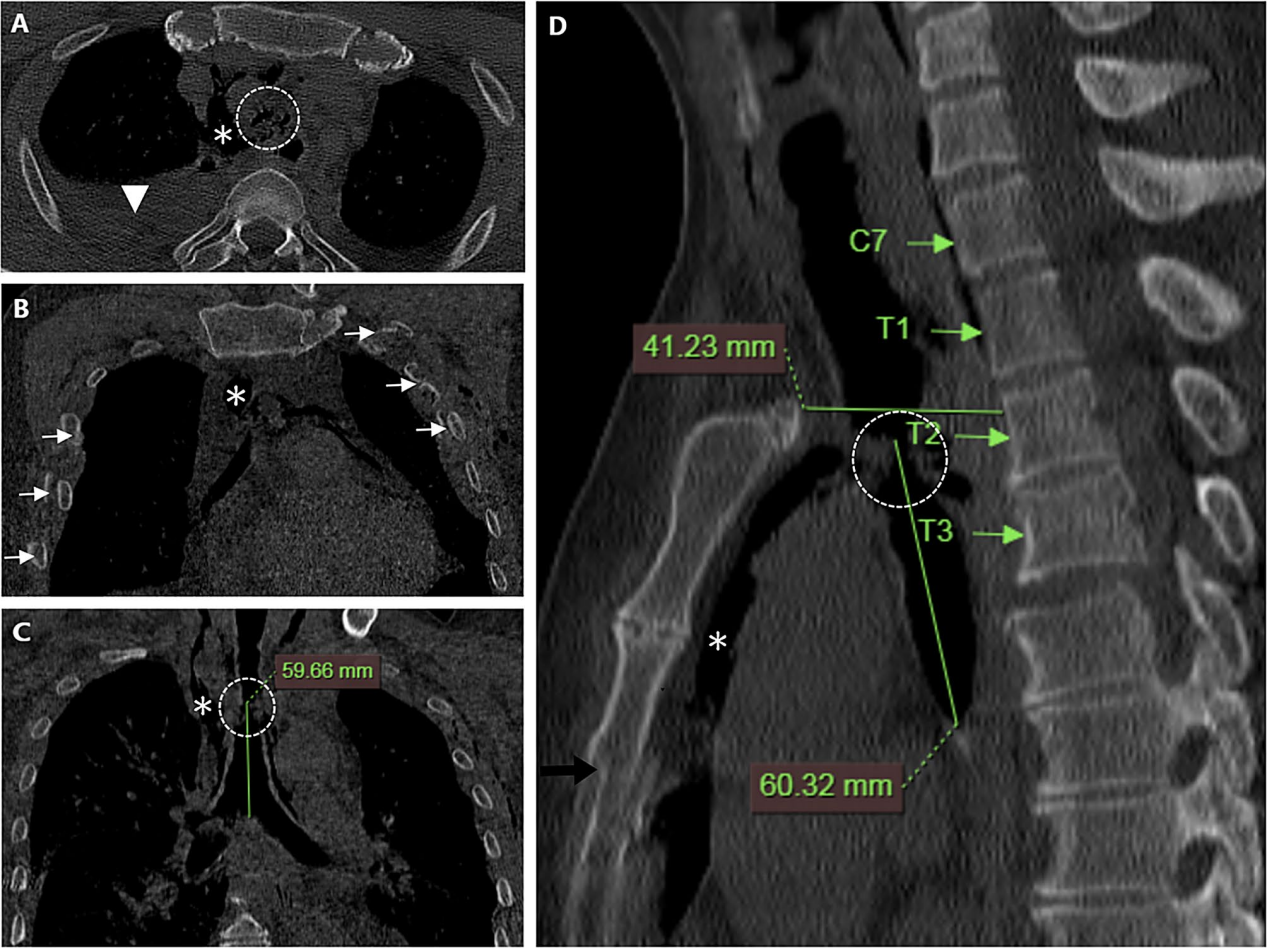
A whole-body CT scan shows a complete tracheal separation 6 cm above the carina (white circle in A, C, D); subcutaneous and mediastinal emphysema (star in A–D); right-sided hemothorax (white arrowhead in A); sternum fracture (black arrow in D); symmetrical bilateral multiple rib fractures (white arrows in B); and posterior dislocation of the T3 vertebra (arrow with T3 in D). (D) The posterior displacement of vertebrae C7 to T3; the shortest distance from the manubrium to the vertebral body is 4.1 cm
